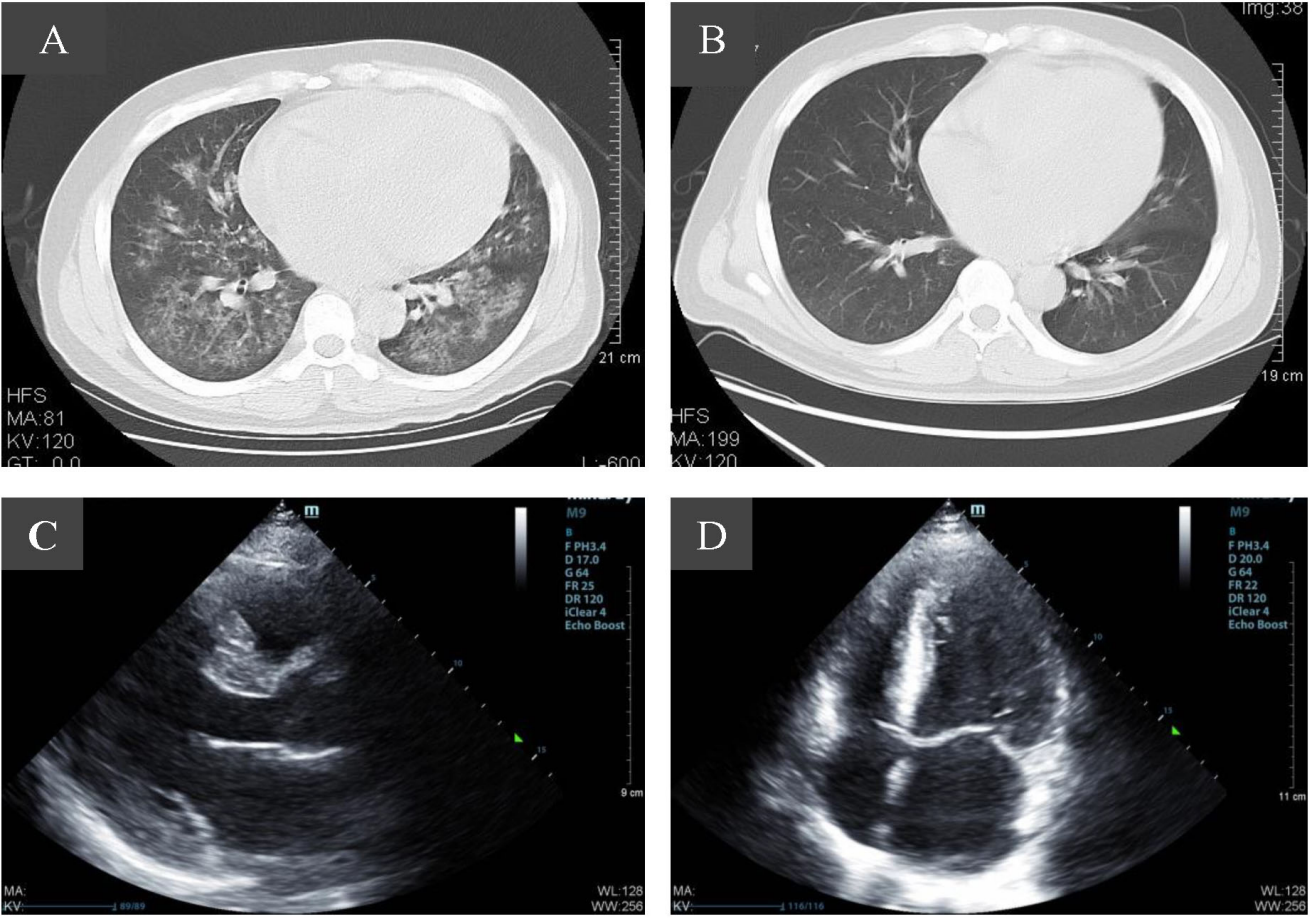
Imaging changes of patients before and after admission. (A) Pulmonary CT scan at admission showed evidence consistent with viral pneumonia combined with pulmonary edema. (B) Ten days after admission, pulmonary infection was significantly reduced. (C,D) Color Doppler echocardiography showed an enlarged heart size with a large left atrium and left ventricular hypertrophy.
